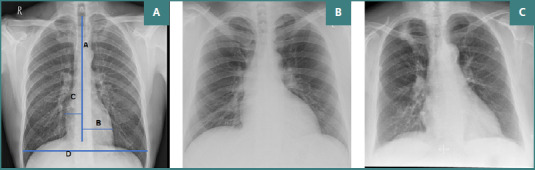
Chest radiographs and cardiothoracic ratio measurements. A, Chest radiograph demonstrating measurements for calculating the cardiothoracic ratio (CTR) in normal size heart of DM patient; B, Chest radiograph of moderate cardiomegaly in DM patient; C, Chest radiograph of severe cardiomegaly in DM patient.
